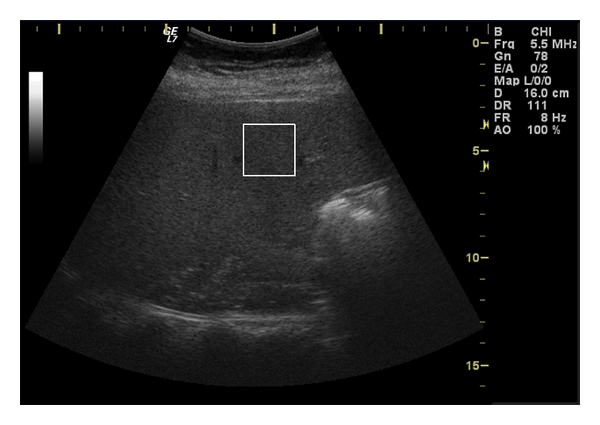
Right lobe ultrasound image. White square represents the region of interest.
